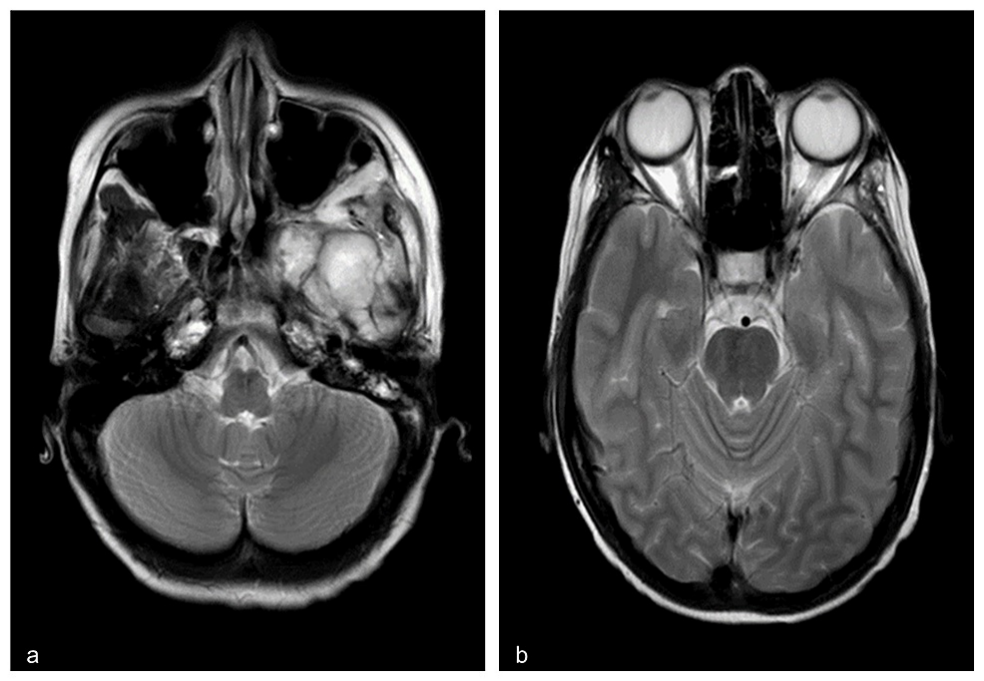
T2-weighted axial MRI brain a) Demonstrating heterogeneously hyperintense mass in left masticator space with locoregional involvement and extension, b) Demonstrating no enhancement of optic nerve and intruded of the tumour to posterior orbit.
